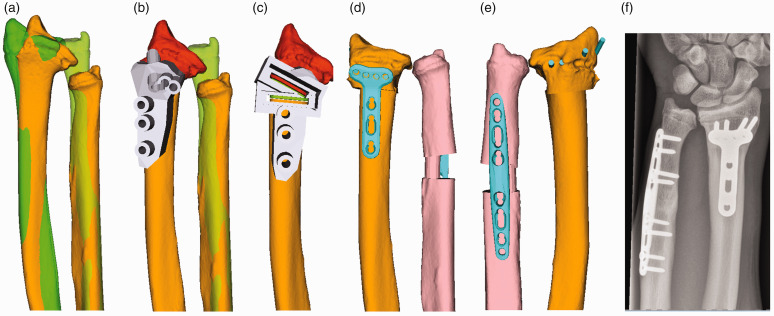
Post-traumatic severe ulnar-minus variance with preoperative computer-assisted, three-dimensional planning of the radius osteotomy, distal radioulnar joint (DRUJ) reconstruction and ulnar lengthening. (a) Three-dimensional bone model of pathologic DRUJ (orange), uninjured contralateral side (green), (b) drill guide (grey) and distal part of the radius (red), (c) saw guide to remove a bone wedge, (d) anterior view of the reconstructed DRUJ with distal radius plate and long ulnar shortening plate (mint), (e) dorsal view of the reconstructed DRUJ, (f) postoperative anterior–posterior radiographs of the wrist.
